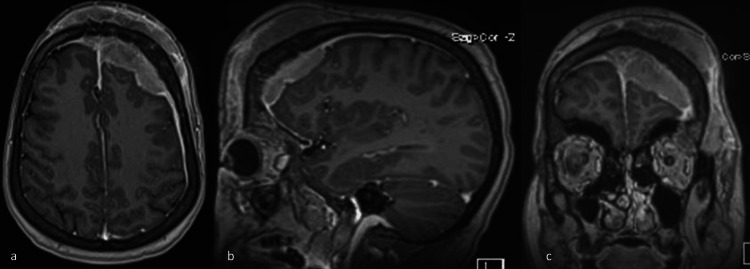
T1 contrasted MRI [a] axial, [b] saggittal, and [c] coronal images showing a frontal, extra-axial, enhancing lesion, extra-axial enhancing lesions, with extension to the overlying subcutaneous tissue. The lesion is occupying the superior sagittal sinus in keeping with invasion.
